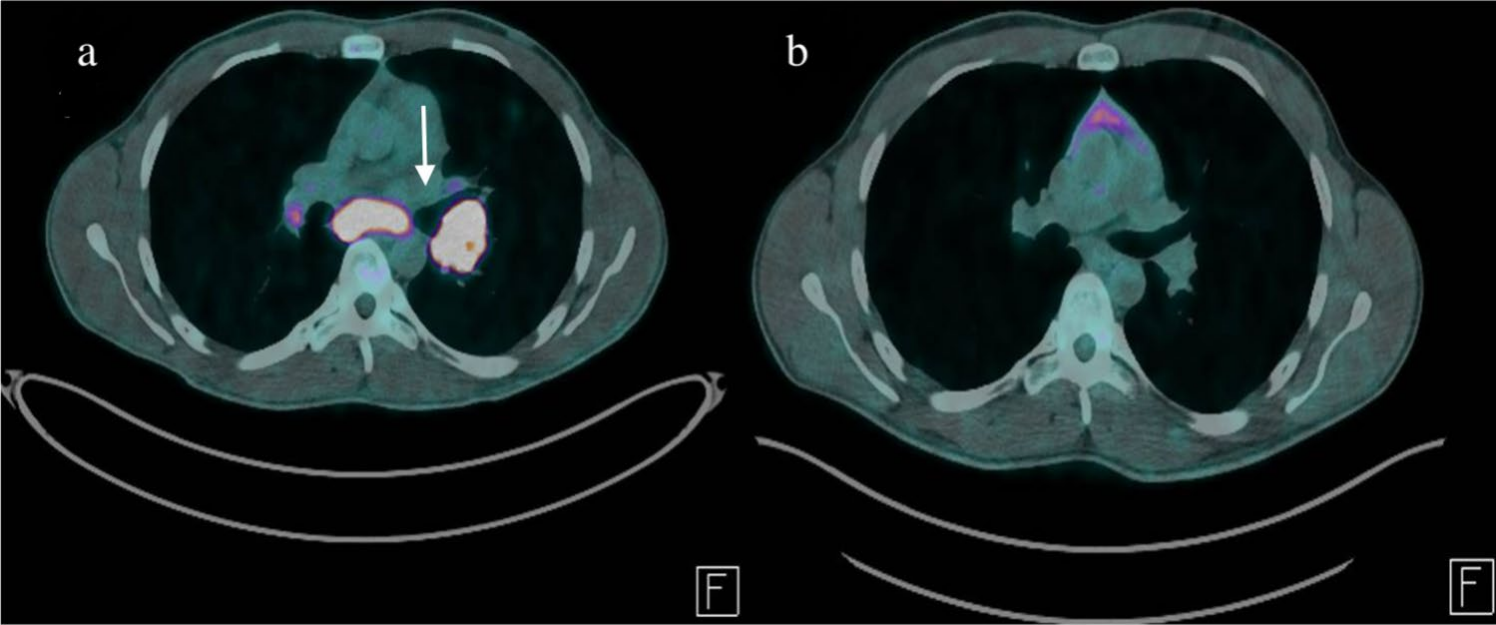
Whole-body 18F-FDG PET scans (a) Baseline scan obtained during patient hospitalization, showing multiple hypermetabolic mediastinal lesions (arrow). (b) Six-month follow-up scan after TNF-α inhibitor withdrawal, showing marked improvement of abnormal FDG uptake
